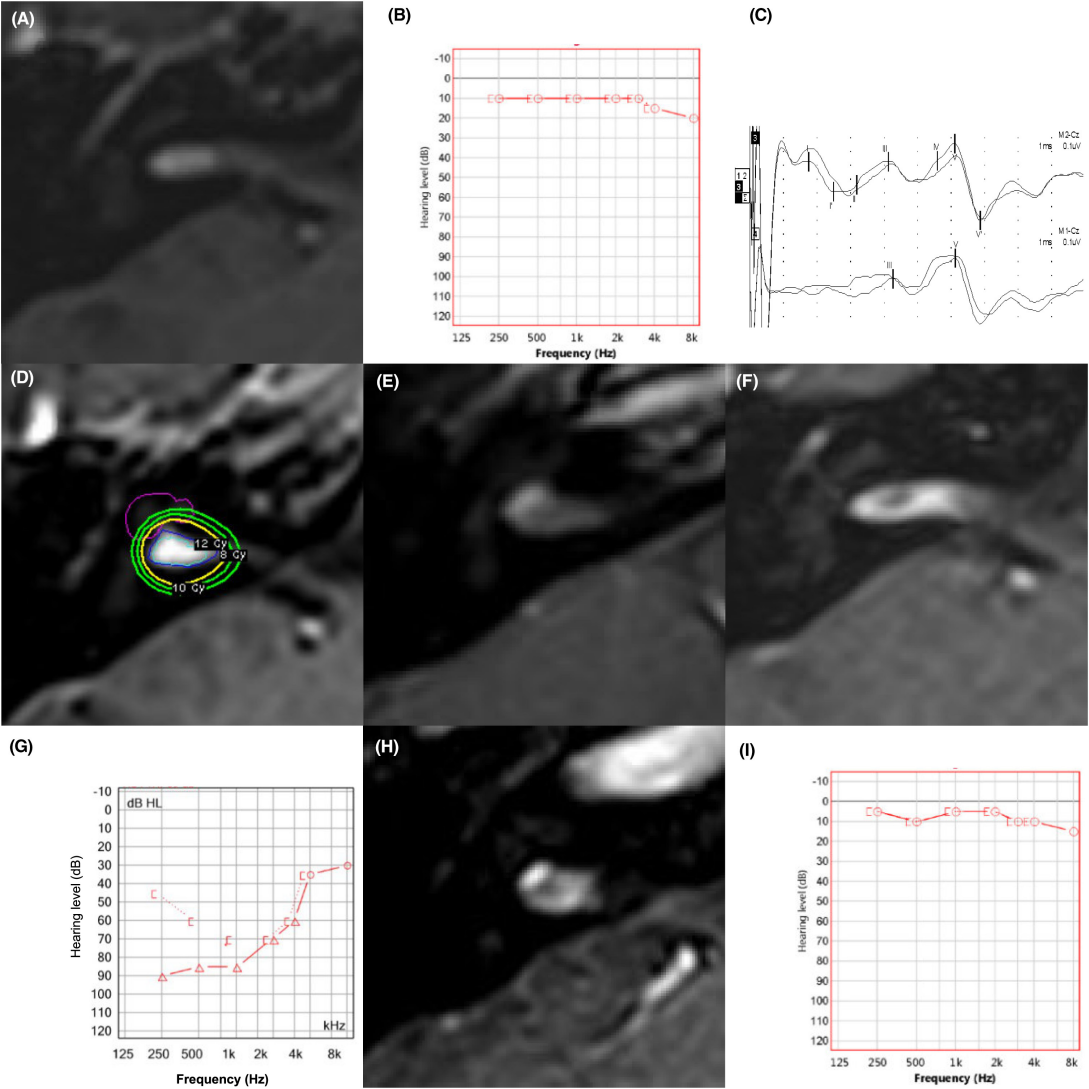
A representative case of a petit vestibular schwannoma. A 38‐year‐old female patient with a petit vestibular schwannoma on the right side with a volume of 0.045 cm^3^ (A). She had serviceable hearing with an average of 11 dB in pure tone audiometry (B), and in the preradiation brain stem auditory evoked potential, the difference in latency between waves I and V was 4.36 ms on the right side (C). Gamma knife radiosurgery was performed using a 4‐mm dual shot with a marginal dose of 12 Gy (D). Six days after radiosurgery, the tumor showed the minimal change in MRI taken due to neck discomfort (E). The volume of the tumor increased to 0.056 cm^3^ at 6 months after radiosurgery (F), and hearing was deteriorated to the unserviceable range with a PTA of 75 dB (G). Thirty‐one months after radiosurgery, the volume of the tumor decreased (H), and the average PTA improved to 7 dB (I).
